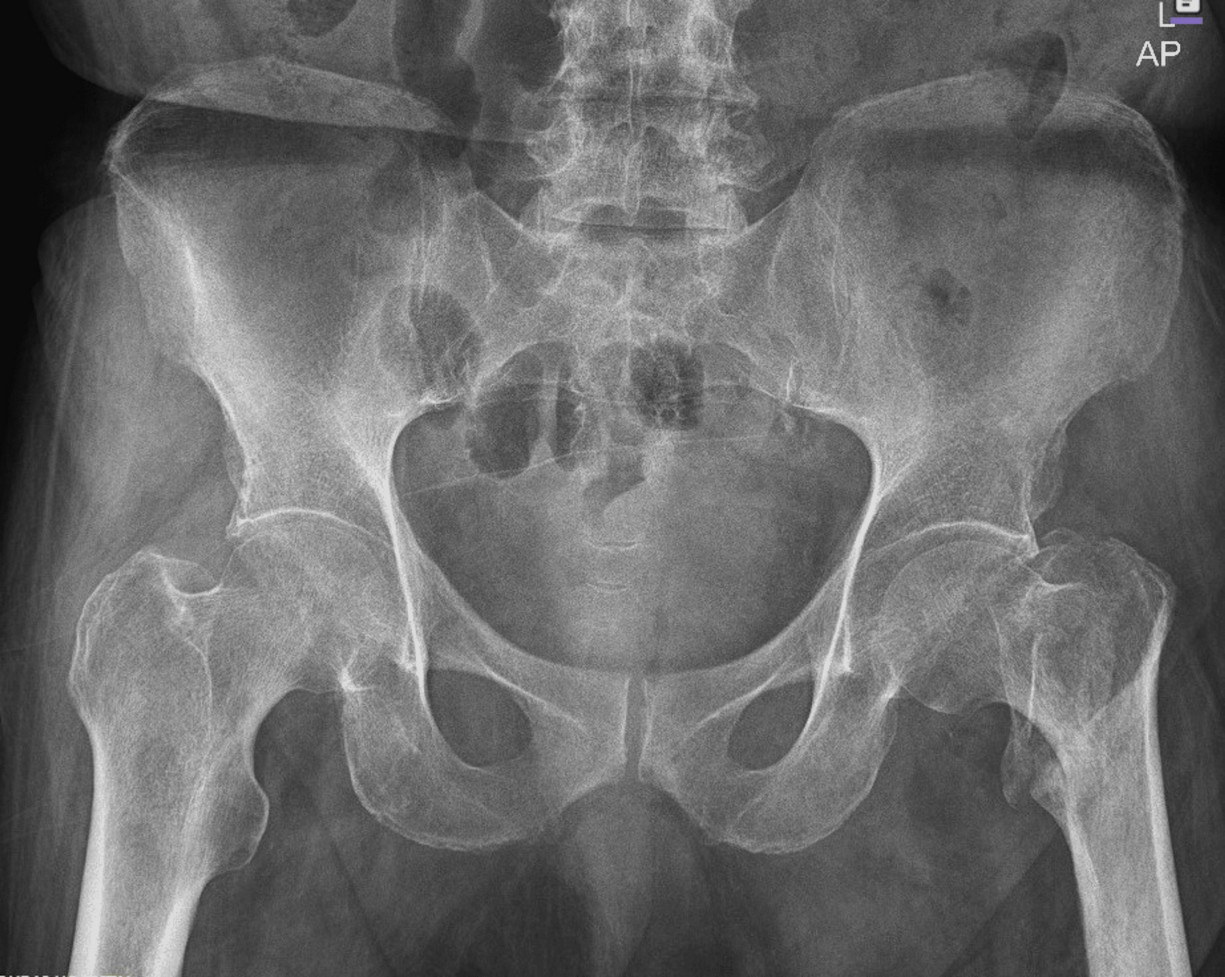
X-ray pelvis showing the mildly displaced intertrochanteric fracture of the left femur.
